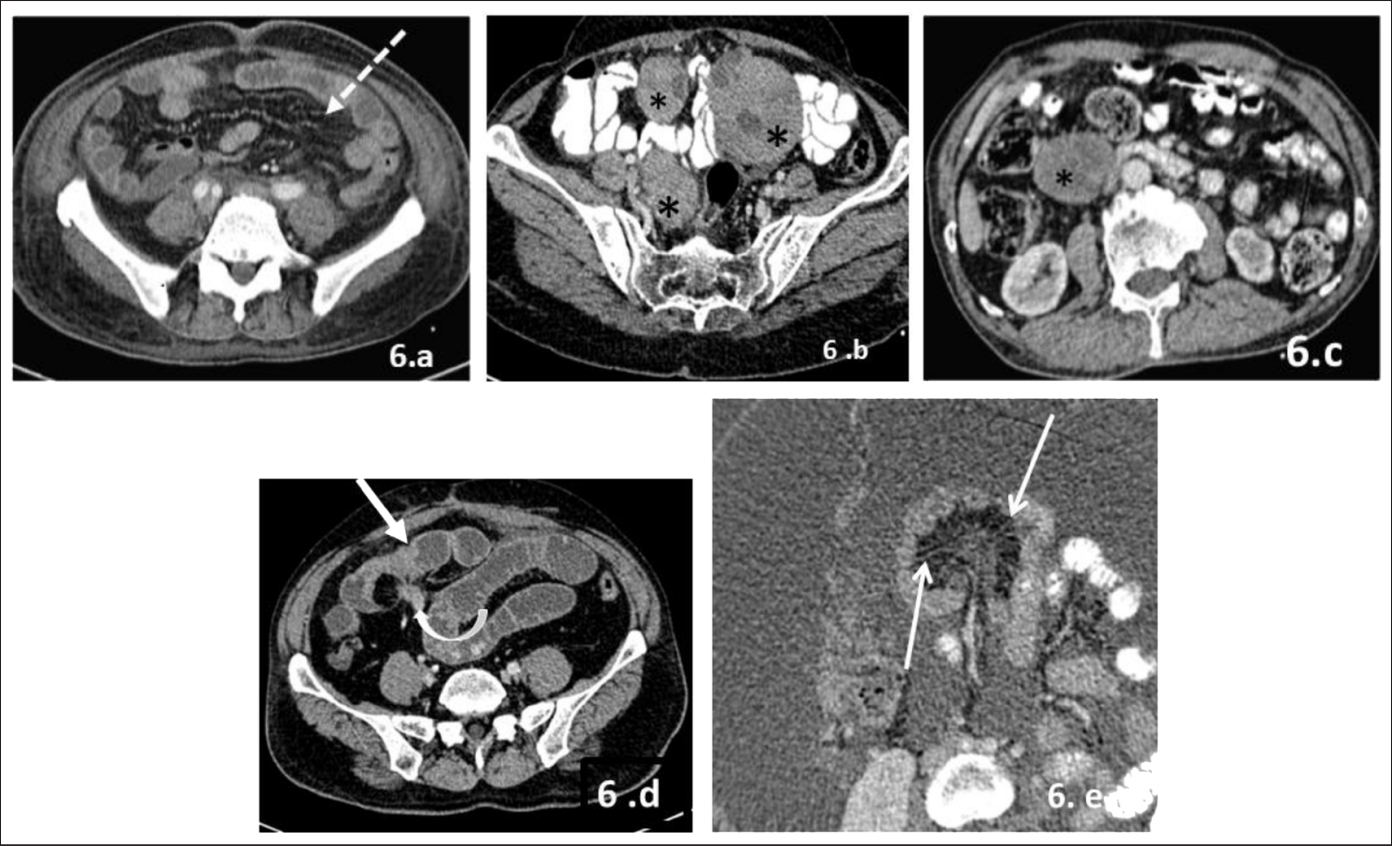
(a–e) Patterns of involvement of mesentery in peritoneal carcinomatosis. 1) Diffuse mesenteric fat stranding (dotted arrows in a). 2) Nodules/masses: CECT images of the abdomen in patient with disseminated granulosa cell tumour of ovaries (b) reveal multiple solid cystic masses in mesentery (asterisk). CT scan in another patient with bronchogenic carcinoma (c) reveal soft tissue deposit in mesentery (asterisk). 3) Mass contiguous with small bowel thickening/mass: CECT image of the abdomen in a patient with ileal carcinoid reveal ill-defined enhancing wall thickening in terminal ileal loops along with spiculated mass in adjacent mesentery (d). 4) Stellate mesentery: Axial contrast enhanced CT images (e) of the abdomen in a patient with ovarian carcinoma reveal gross ascites, ill-defined stranding in mesentery along mesenteric vessels resulting in thickening and rigidity of mesentery and straightening of mesenteric vessels (thin white arrows).
